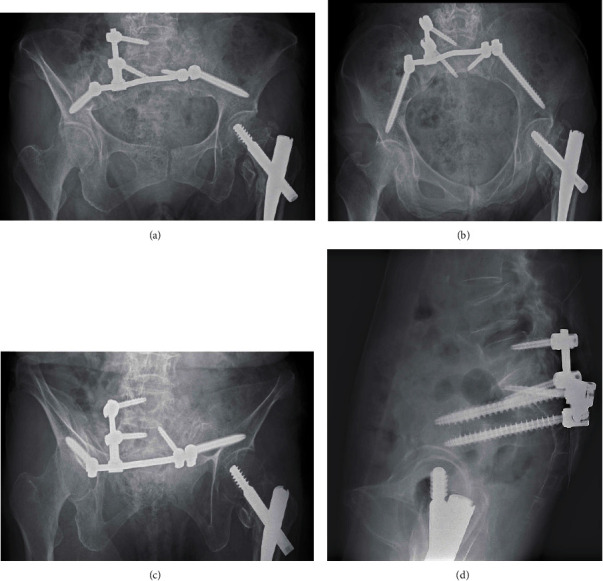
Postoperative X-ray of anteroposterior, inlet view, outlet view, and lateral view.
